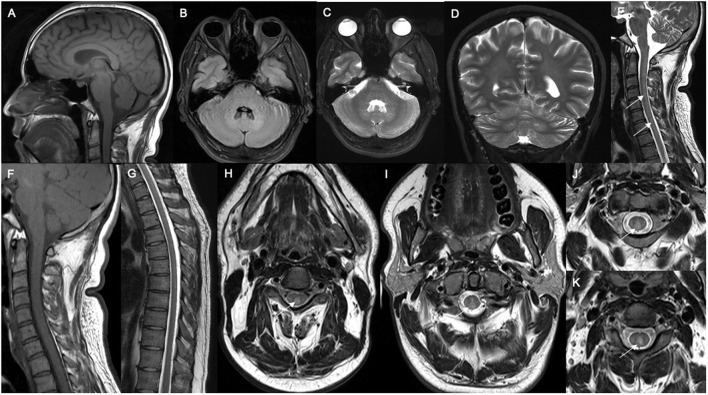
Neuroradiological features in spinal CTX. (A) Sagittal brain MRI showed normal cerebellar and corpus callosum structure. Axial brain MRI showed mild hyperintensity in dentate nucleus in FLAIR (B) and T2W sequences (C), as well as hyperintensity in dentate nucleus in coronal T2W imaging (D). Sagittal spine MRI showed hyperintense (white arrows) in T2W imaging (E) and isointense change in the dorsal column of the cervical spinal cord in T1-weighted imaging (F). Sagittal thoracic spine MRI showed normal structure (G). Axial cervical spine MRI showed marked hyperintensity of the dorsal column (white arrows) in T1W (H) and T2W imaging (I–K).
